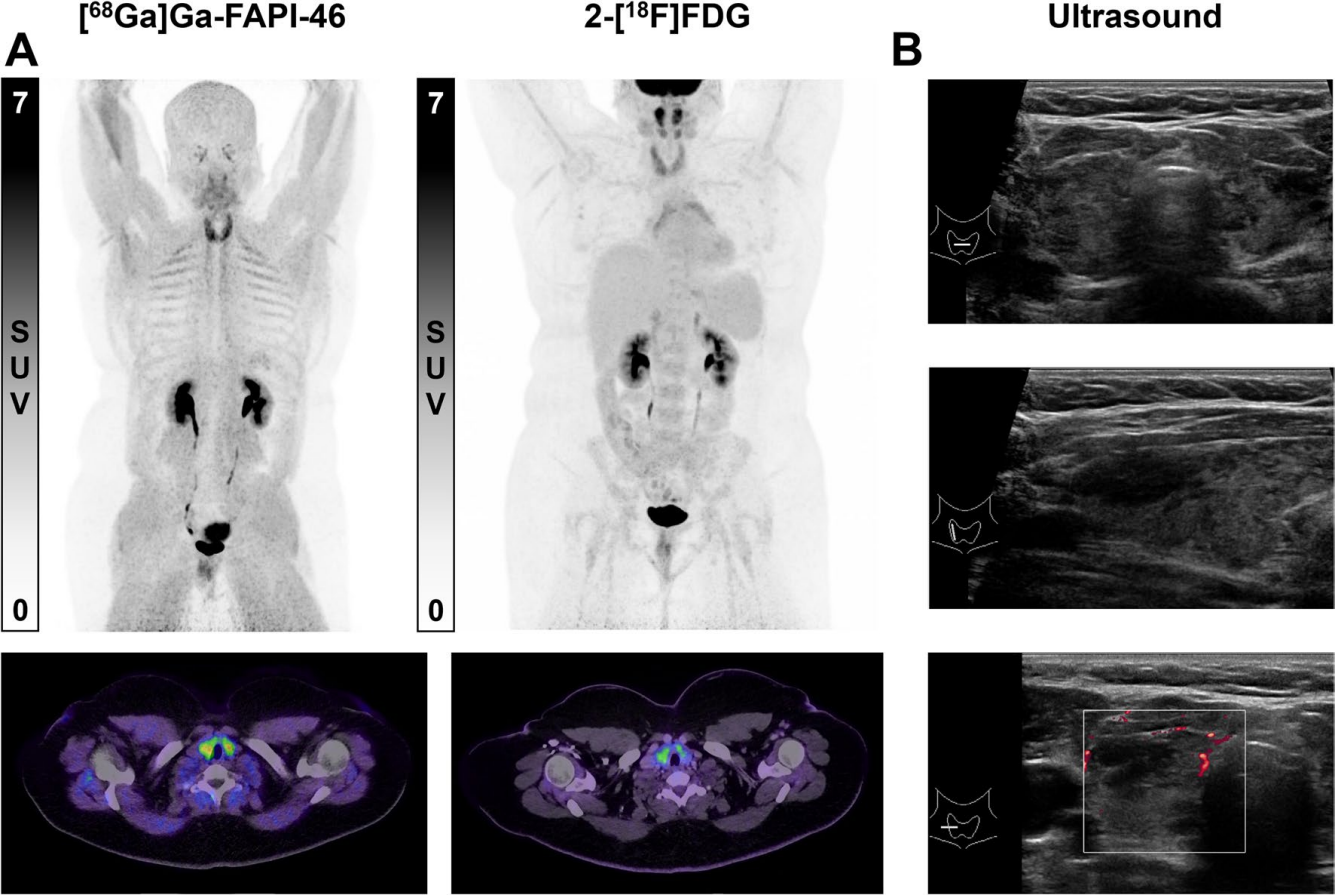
Example of thyroid uptake on [^68^Ga]Ga-FAPI-46 and 2-[^18^F]FDG PET in patient no. 3. A shows the maximum intensity projection of [^68^Ga]Ga-FAPI-46 and 2-[^18^F]FDG PET/CT and axial images of the thyroid. B shows ultrasound findings of the thyroid with inhomogeneous parenchyma and normal perfusion
